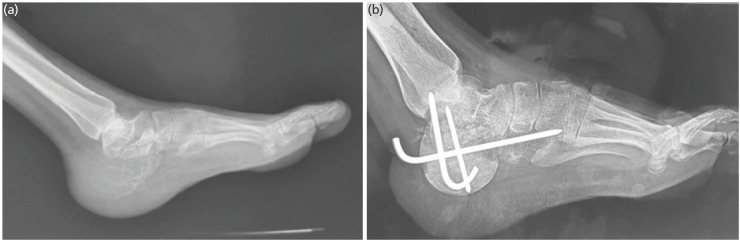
a) Lateral view of the left foot showing an expansile lytic lesion of the calcaneum with no visible cortical margin posteriorly; Campanacci Grade 3. (b) Post-operative radiograph, lateral view, at two weeks after removal of stitches, with femoral head allograft implanted after resection and fixation with Kirschner.
